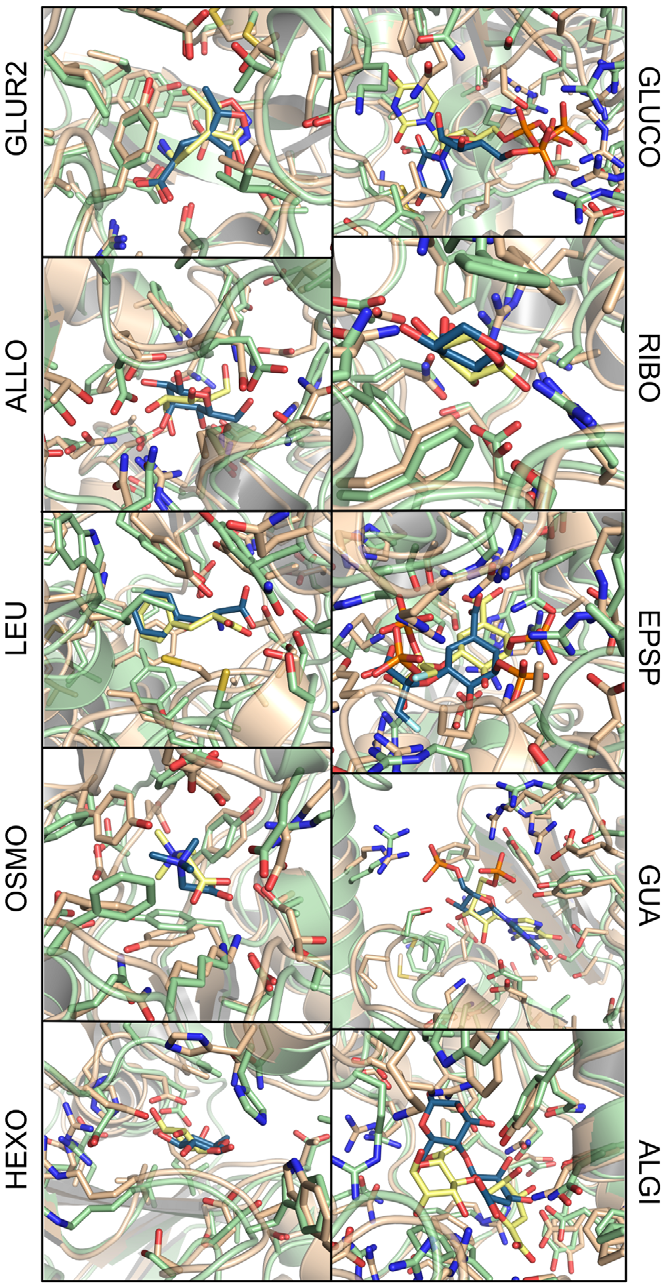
Modeled protein/ligand complexes. X-ray structures of receptor and ligand are shown in wheat and yellow, modeled complexes are colored green/blue.
